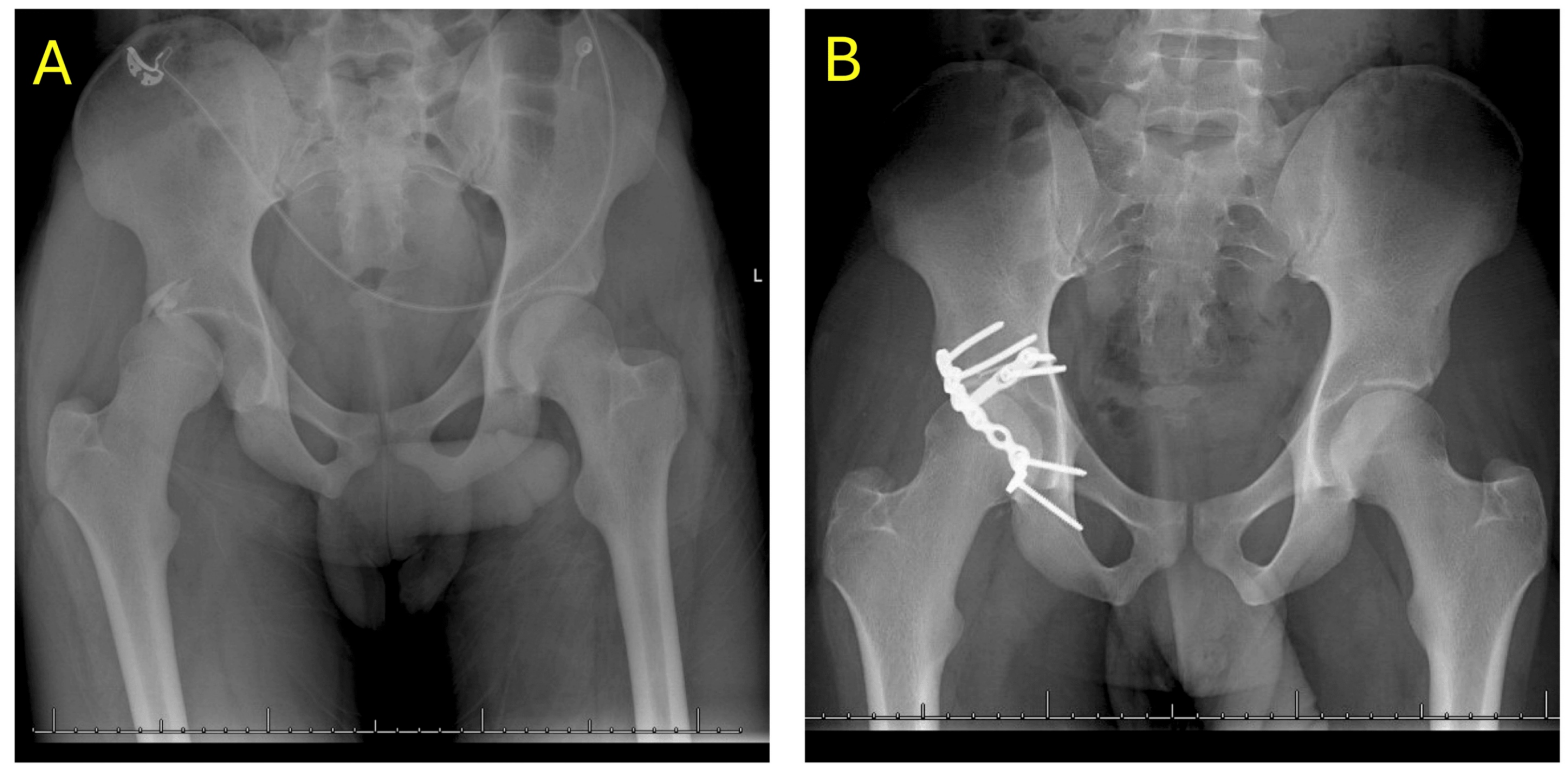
Serial radiographs of subject 5. (A) Injury film demonstrating initial fracture pattern. (B) Radiograph obtained 174 days post-operatively demonstrating interval healing and alignment.
